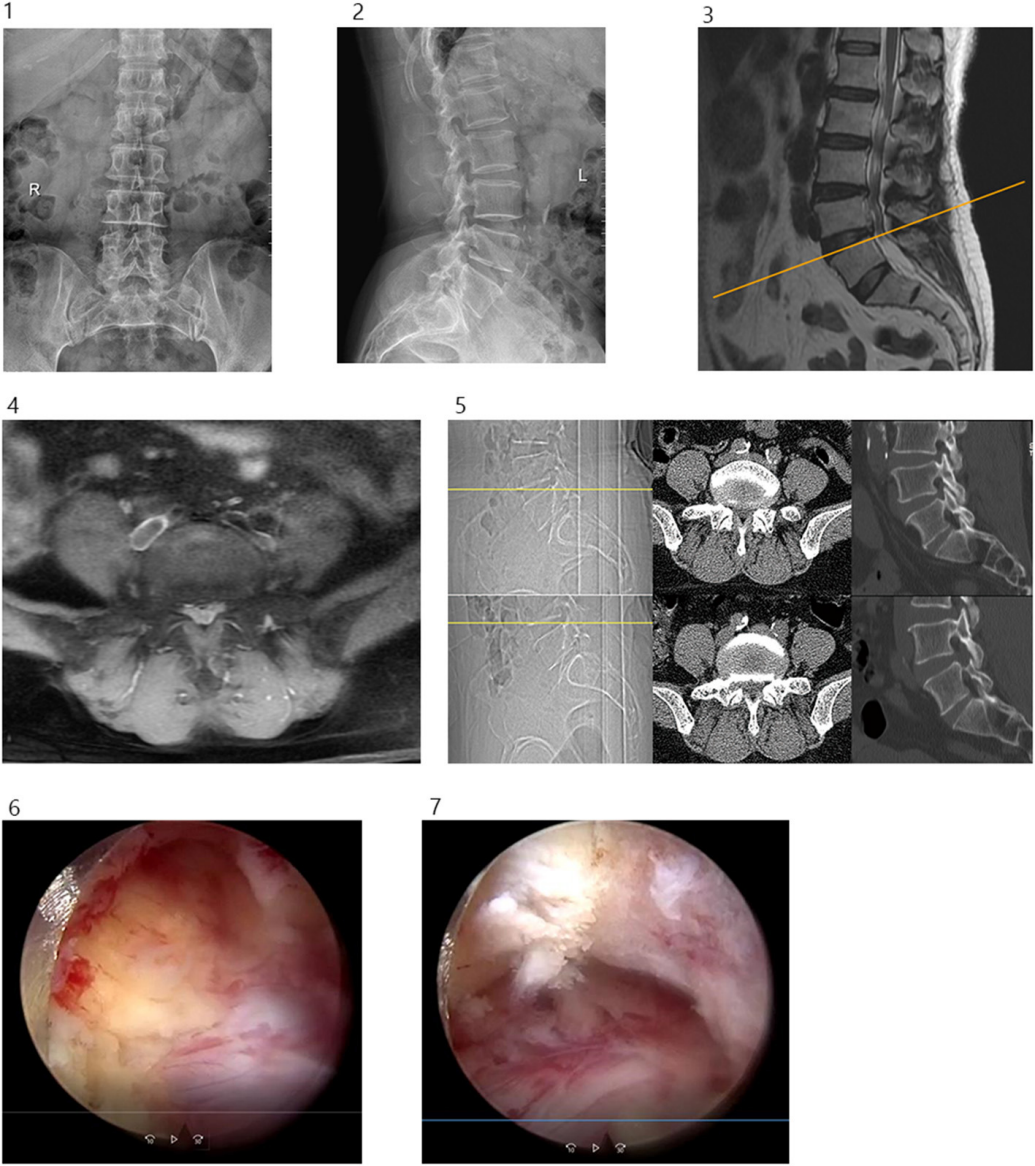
(1) Preoperative lumbar spine anteroposterior projection. (2) Preoperative lumbar spine lateral position. (3 and 4) Preoperative lumbar spine MR showed lumbar 4/5 disc herniation with spinal stenosis. (5) Before and after operation, CT cross-sectional image showed lumbar 4/5 decompression of the right spinal canal, sagittal reconstruction showed partial ventral resection of the superior articular process of L5, partial resection of the upper edge of the vertebral pedicle of L5. (6) Ventral partial resection of the superior articular process of L5 was observed under endoscopy. (7) Partial resection of the upper edge of the pedicle of L5 was observed under endoscopy.
